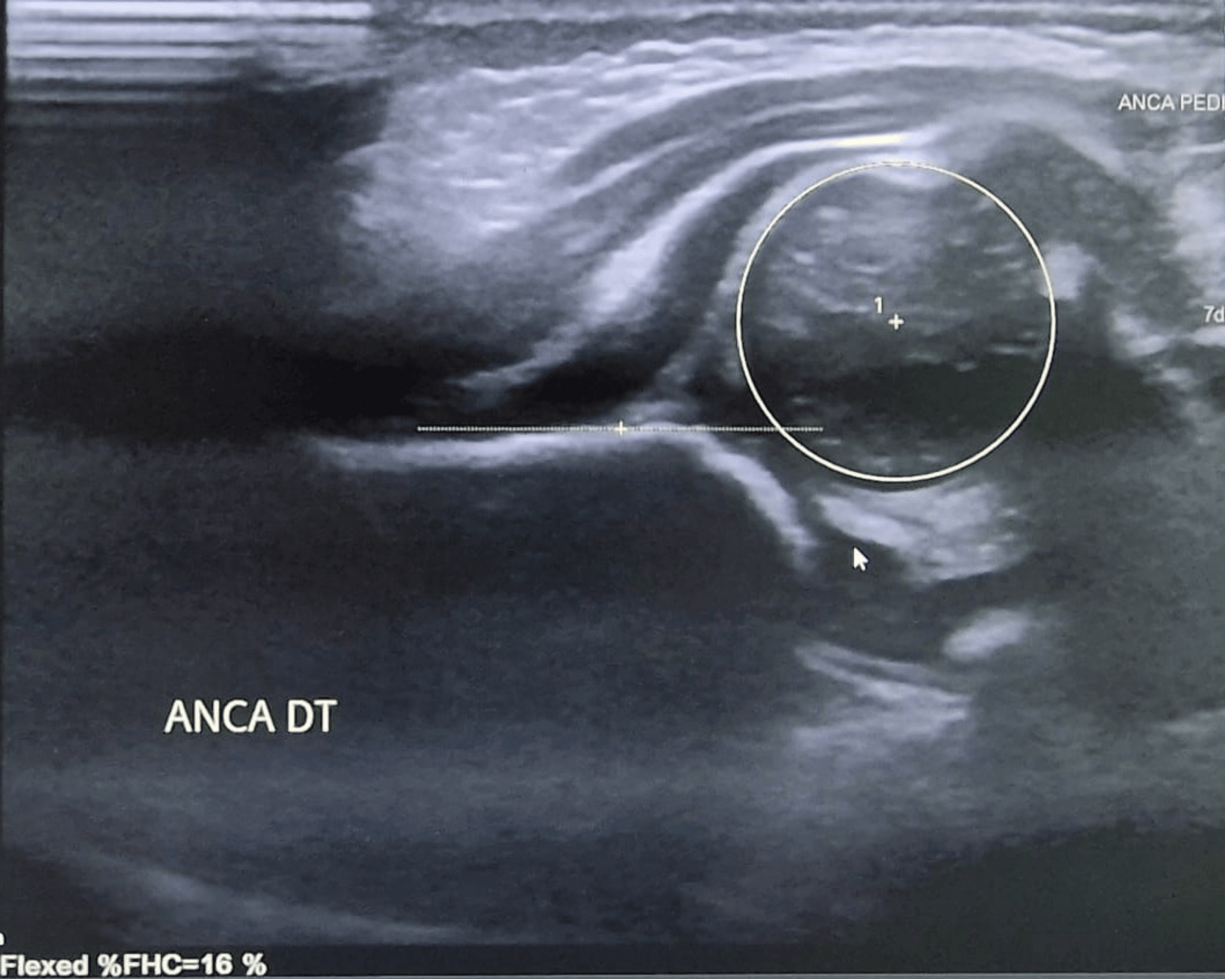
Hip ultrasound at six weeks of age showing right-sided developmental dysplasia of the hip (DDH), with femoral head coverage (FHC) of 16% in flexion
